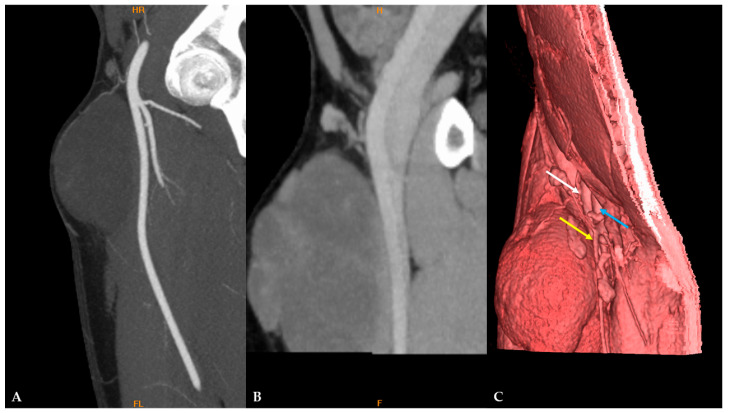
CT angiography reconstructions show the relationships between the MLS and adjacent femoral arteries and veins: (A,B) no signs of infiltration of CFA, SFA, and deep femoral veins; (C) 3D image with highlighted vascular structures adjacent to MLS. CFA (white arrow), deep femoral vein (blue arrow), and great saphenous vein (yellow arrow).
